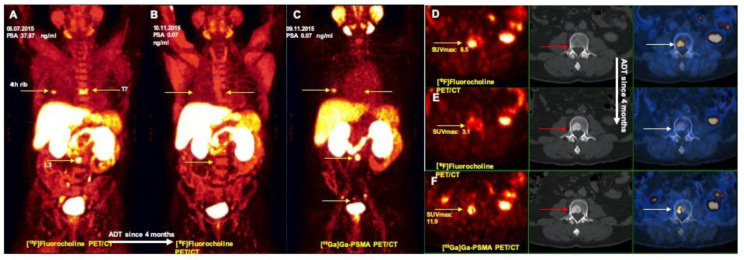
Prostate cancer treatment monitoring: more accurate evaluation of the treatment by [^68^Ga]Ga-PSMA PET/CT. (A) [^18^F]Fluorocholine PET: MIP revealed multiple bone metastases (arrows) on 4th right rib, T7 and L3 vertebra corresponding with morphological changes on CT. (B) Treatment monitoring by [^18^F]Fluorocholine PET: MIP showed no abnormal tracer uptake in the known metastases (arrows), suggestive complete response well correlating with PSA value of 0.07 ng/mL. (C) Treatment monitoring by [^68^Ga]Ga-PSMA PET MIP revealed still metabolic activity on the 4th right rib, and L3 vertebra (arrows) showing clear superiority to [^18^F]Fluorocholine PET and clinical findings. (D) transaxial views: PET (left), CT (middle) and fusion PET/CT (right) images. (E) transaxial views; PET (left), CT (middle) and fusion PET/CT (right) images. (F) transaxial views: PET (left), CT (middle) and fusion PET/CT (right) images.
